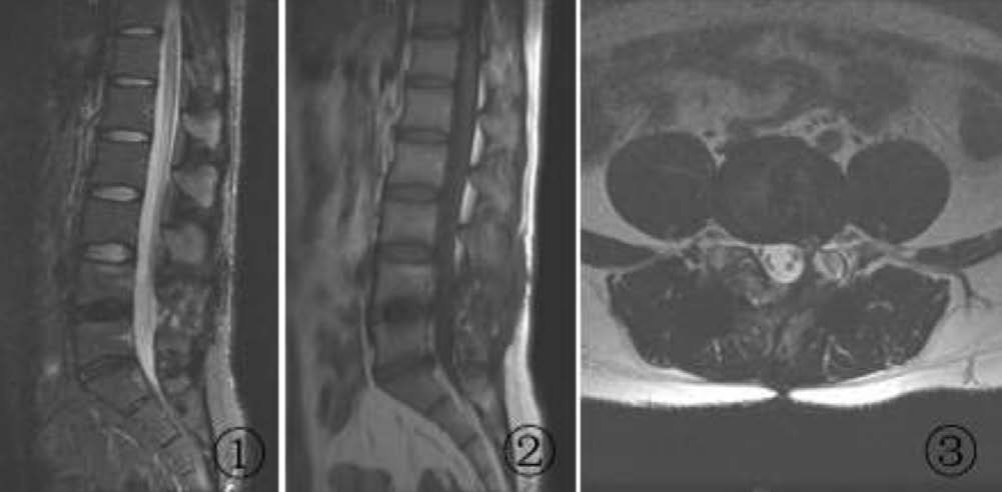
MRI after posterior instrumented lumbar fusion and cyst removal. A (A) sagittal T2-weighted image, (B) sagittal T1-weighted image, and (C) transverse T2-weighted image show the disappearance of the pseudocyst and no significant compression of the left L5 nerve root. MRI = magnetic resonance imaging.
